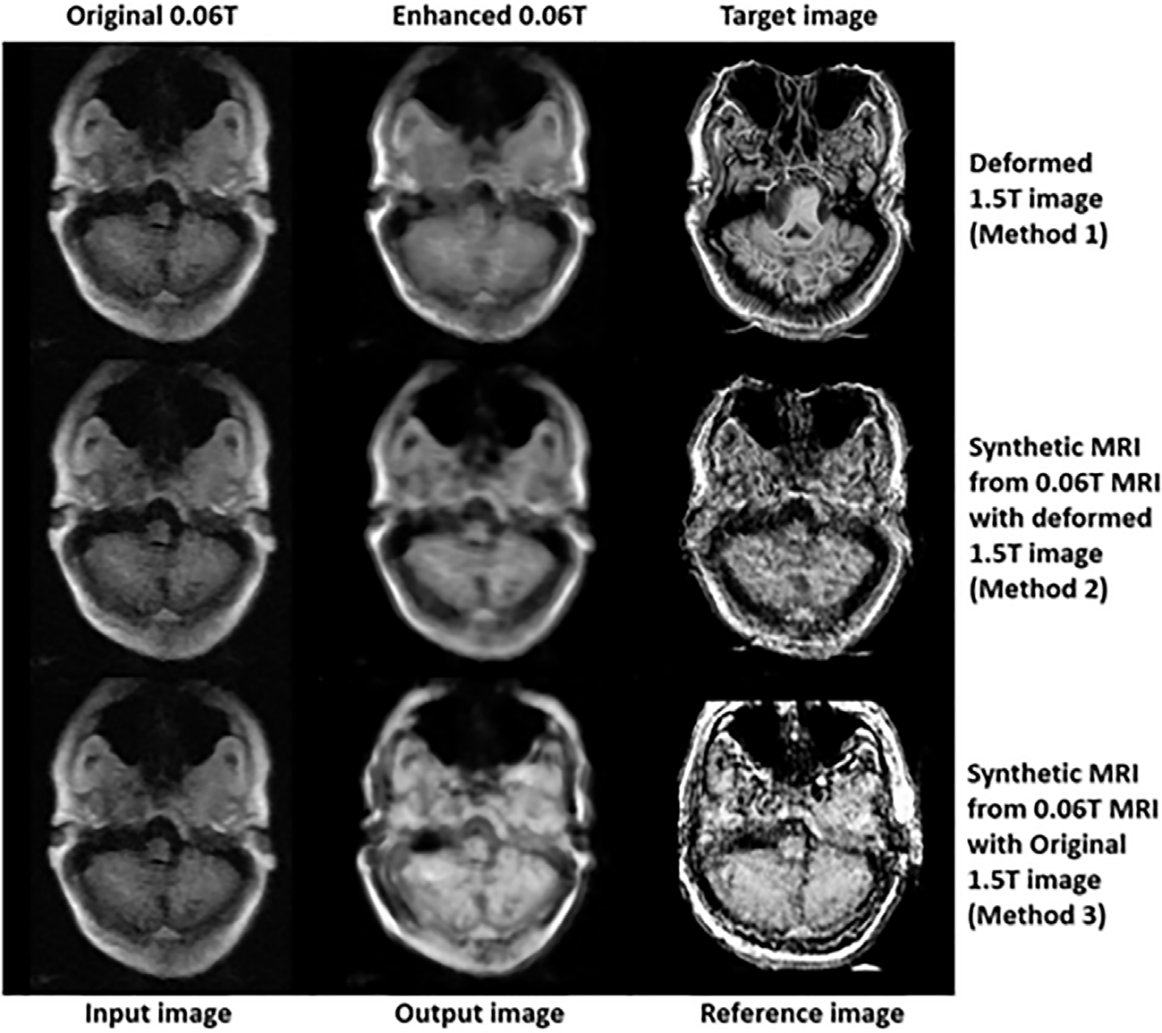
Results of enhanced MRI of slice no. 5 in step 3 (conventional-GAN model).
